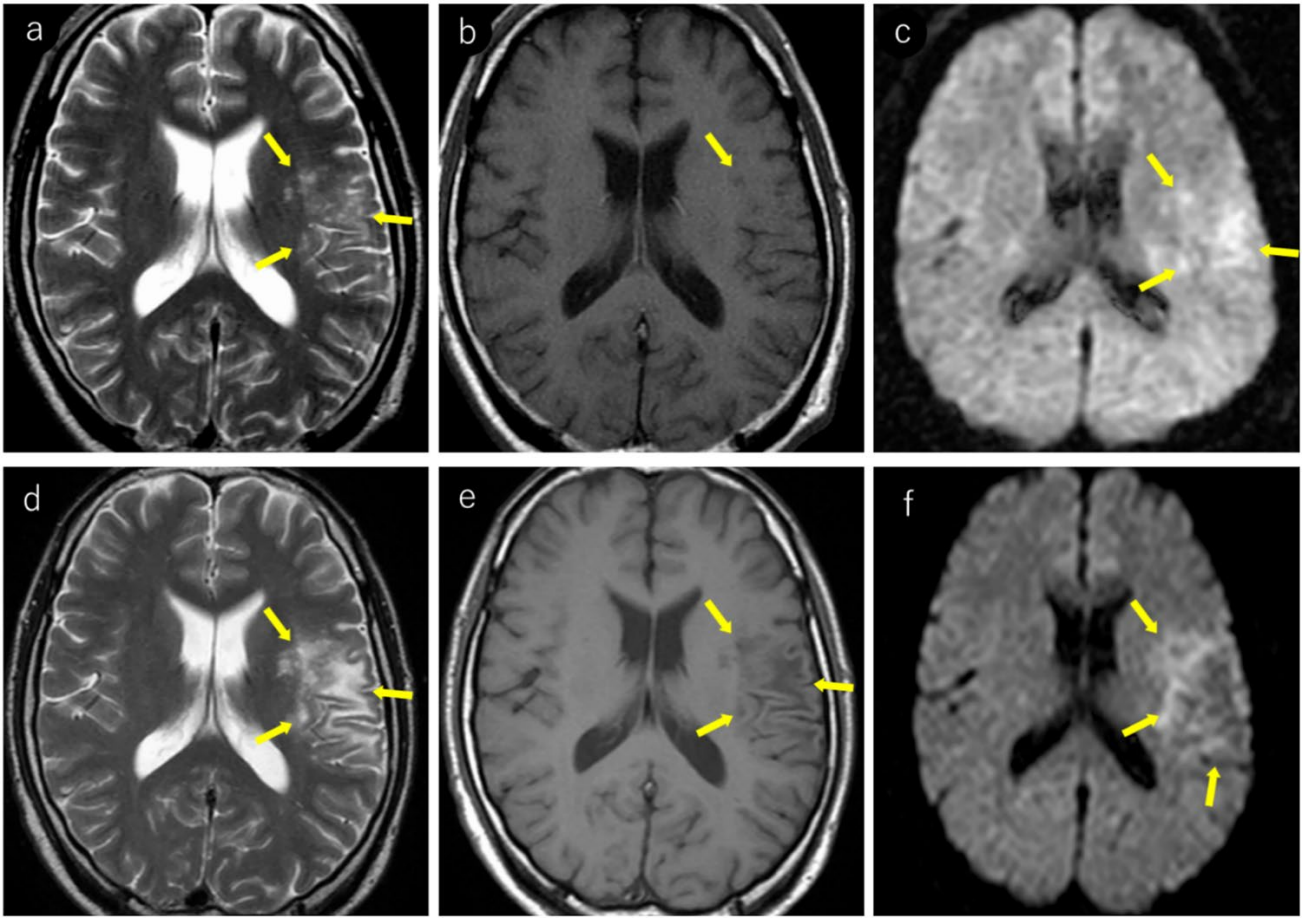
HIV-positive man in his 40 s underwent brain MRI due to weight loss and general fatigue. Initial MRI findings (a–c): a T2-weighted imaging showing punctate hyperintense lesions in the subcortical white matter, primarily affecting the left operculum and insula (yellow arrows). b Some lesions demonstrate subtle hypointensity on T1-weighted images (yellow arrow). c T2 hyperintense areas show corresponding hyperintensities on diffusion-weighted imaging (yellow arrows). PCR testing for JCV in the CSF was negative. After the onset of progressive articulation disorder and decreased muscle strength in the right upper limb, a repeat MRI was performed after 2 months. Follow-up MRI after 2 months (d–f): d T2-weighted imaging demonstrates progression with expansion of the lesions (yellow arrows). e Lesions appear heterogeneously hypointense on T1-weighted images (yellow arrows). f Diffusion-weighted image revealing predominantly peripheral hyperintensities (yellow arrows). A repeat PCR test for CSF JCV confirmed positivity, leading to the diagnosis of PML
